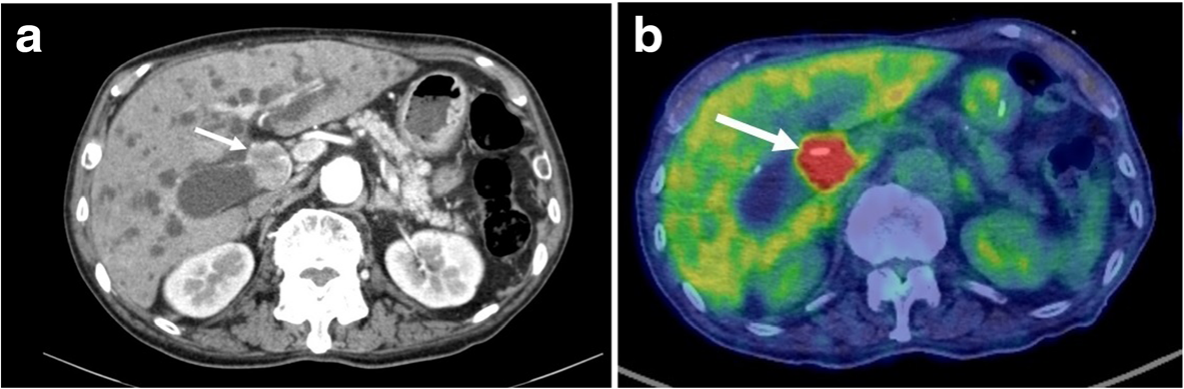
CT (early phase) and FDG-PET findings. a CT showed an enhanced mass that was approximately 2.5 cm in size located in the mid-CBD (*arrow*). b FDG-PET showed a high accumulation of FDG with a maximum standardized uptake value (SUVmax) of 20.7 in the CBD tumor (*arrow*)
